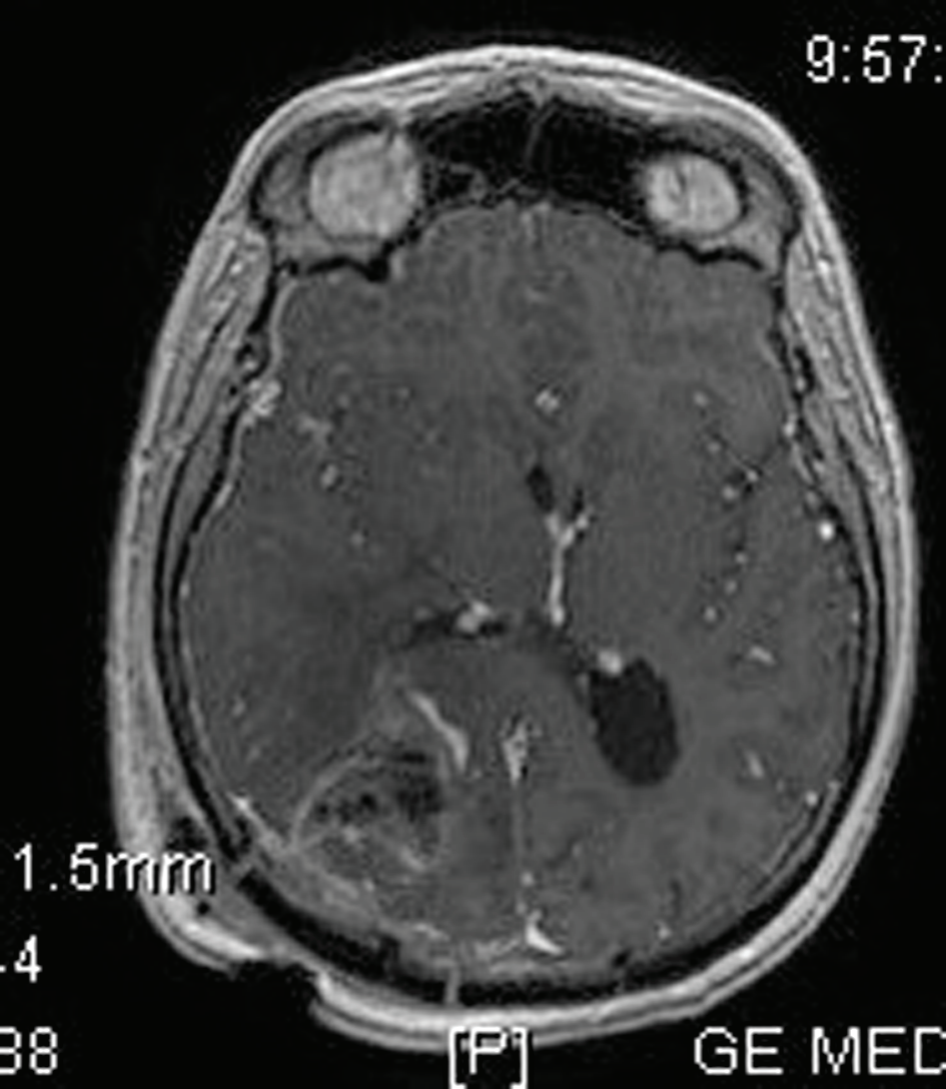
Post-operative brain MRI SPGR imaging which confirms the removal of the tumor.
